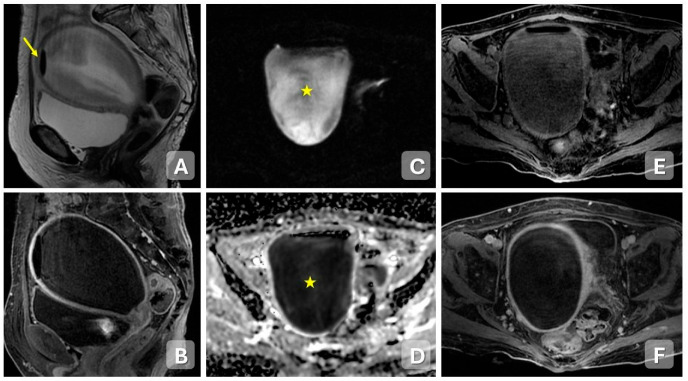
Acute pelvic inflammatory disease—endometritis in a 60-year-old woman with pelvic pain. (A,B) Sagittal T2-weighted and contrast-enhanced T1-weighted MRI images show a distended endometrial cavity filled with fluid containing corpuscular material, and a gas bubble (arrow) at the uterine fundus. Thinning of the myometrium and loss of the junctional zone are also observed. (C,D) DWI and ADC maps demonstrate restricted diffusion (star) within the endometrial cavity, consistent with purulent content. (E,F) Axial fat-saturated T1-weighted images before and after contrast administration show marked post-contrast enhancement of the endometrial lining.
